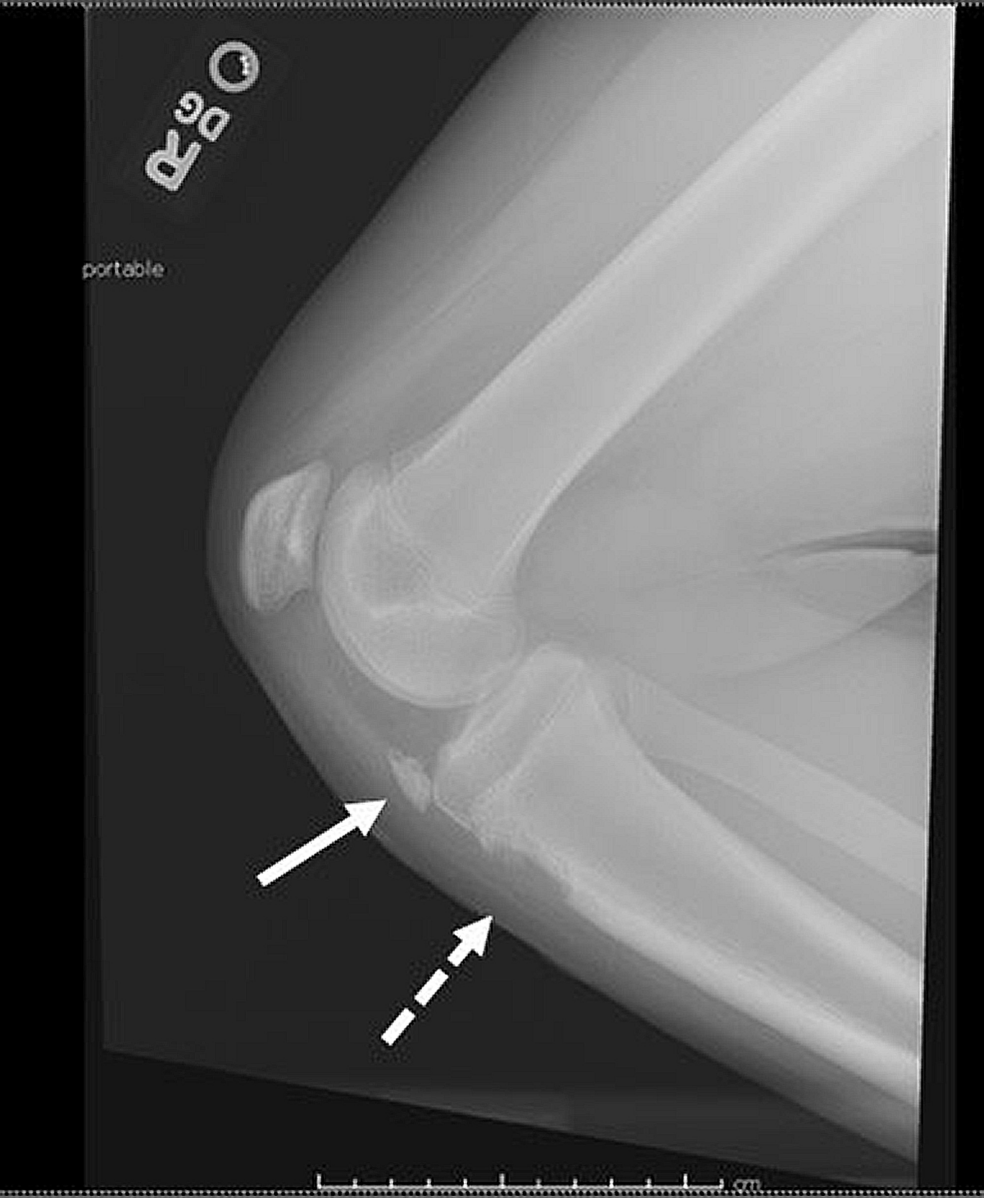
Lateral radiograph demonstrates a 21x9 mm bony fragment (solid arrow) retracted approximately 4.5 cm from the tibial tuberosity (dotted arrow).
